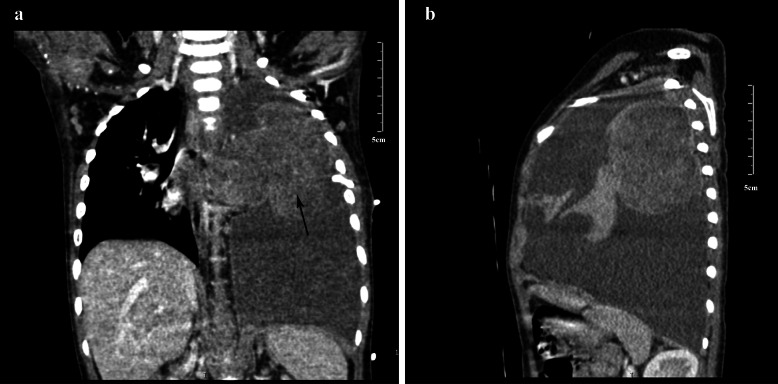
(a and b) Enhanced chest computed tomography (CT) scan revealed 5.9 × 4.8 × 7.6 cm heterogeneous solid lesion, multiple cystic liquefaction foci can be seen inside
